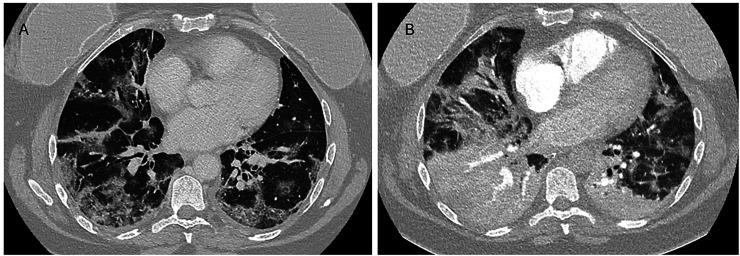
Computed tomography images from patient 1. (a) Day 11 after clinical onset. (b) Day 21 after clinical onset (day of death).
